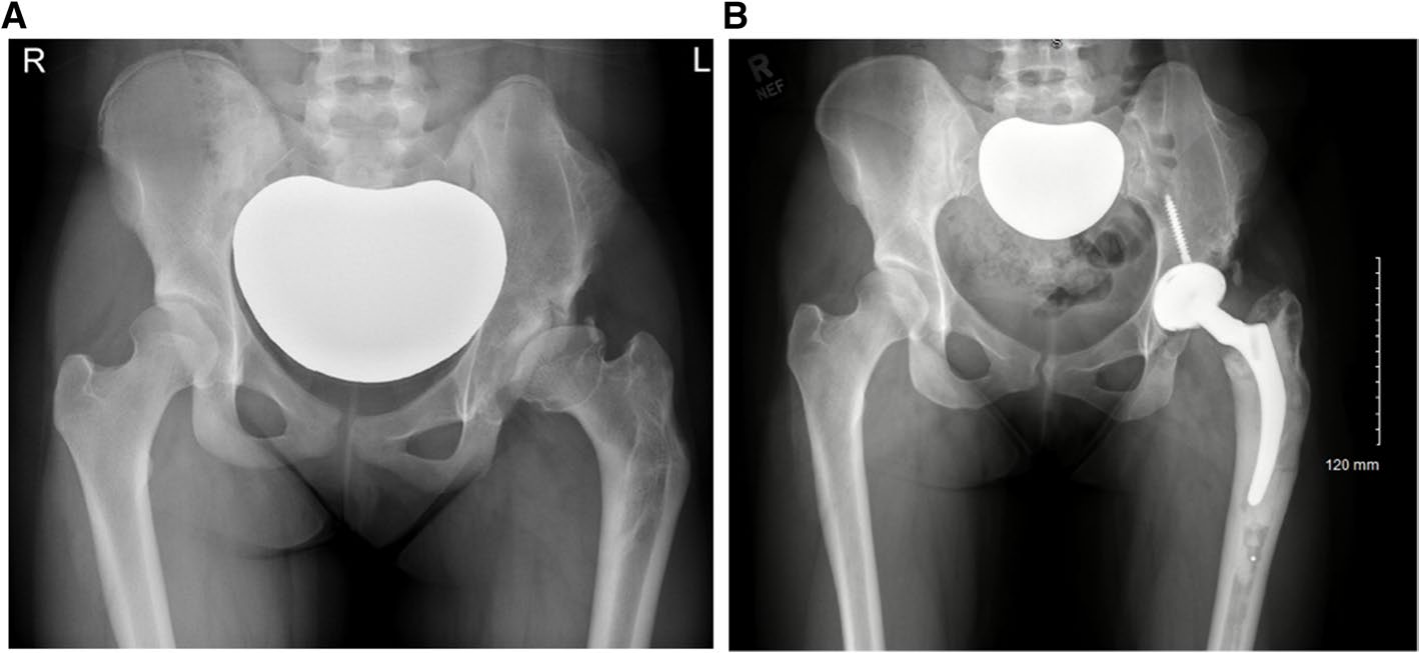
A A pelvic radiograph obtained 15 years after open reduction, Salter innominate osteotomy and femoral varus de-rotation osteotomy. It shows grade III osteonecrosis of the left hip in a 17-year-old girl. There is total femoral head involvement, marked acetabular dysplasia and subluxation of the hip. She had a positive Trendelenburg gait and 1 cm difference in leg lengths. B Pelvic radiograph of the same patient 5 years post THA, performed at age 18 years
